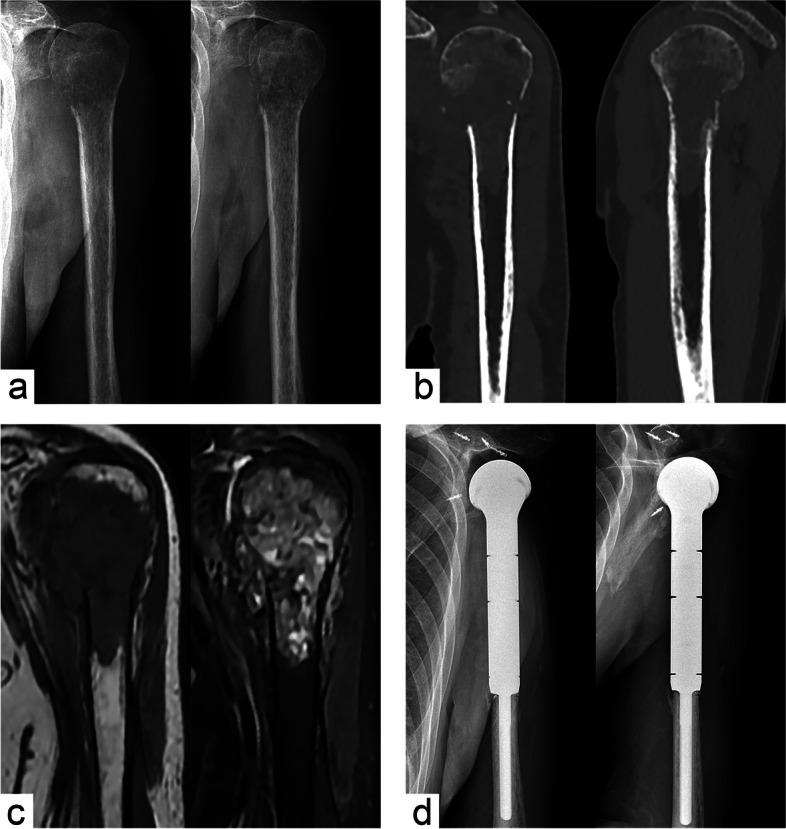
Segmental resection and hemi-shoulder replacement for bone metastases. a Preoperative anteroposterior and lateral X-rays indicated an osteolytic lesion. b CT scan indicated extensive bone erosion and pathological fracture. c MRI suggested that the lesion involved surrounding soft tissues. d Postoperative anteroposterior and lateral X-rays showed that the prosthesis was securely fixed and the joint was stable
